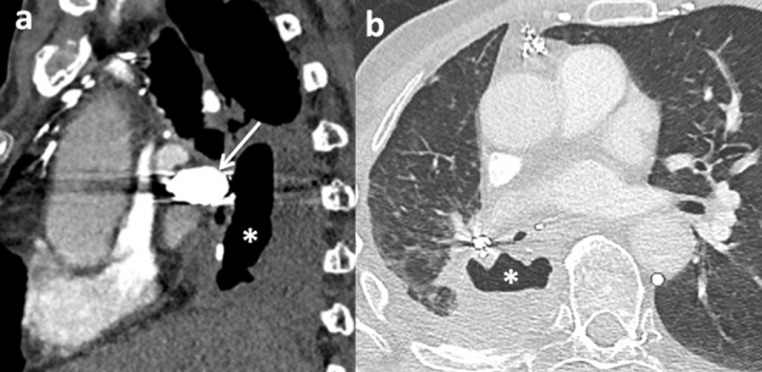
Chest computed tomography (CT) scans with arterial time of injection, 1 month after the second procedure, (sagittal section in mediastinal window (a) and axial section in parenchymal window (b)), show a regression in size and liquid component of the cavity (*), with coil packing of the aneurysmal neck (white arrow).
